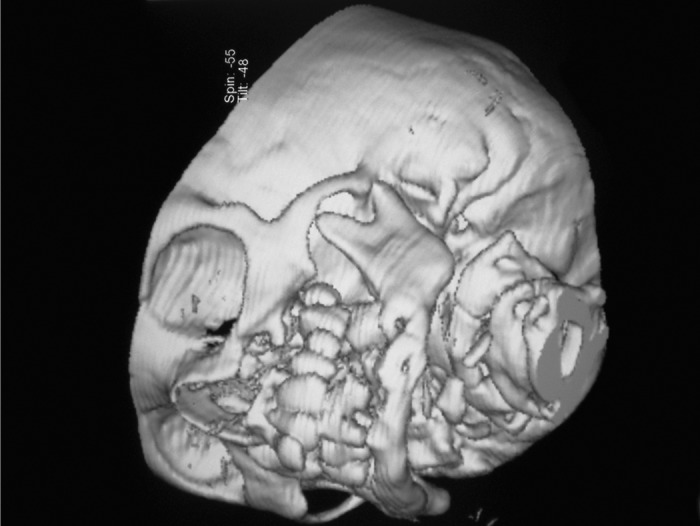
3D view of base of skull CT-image
